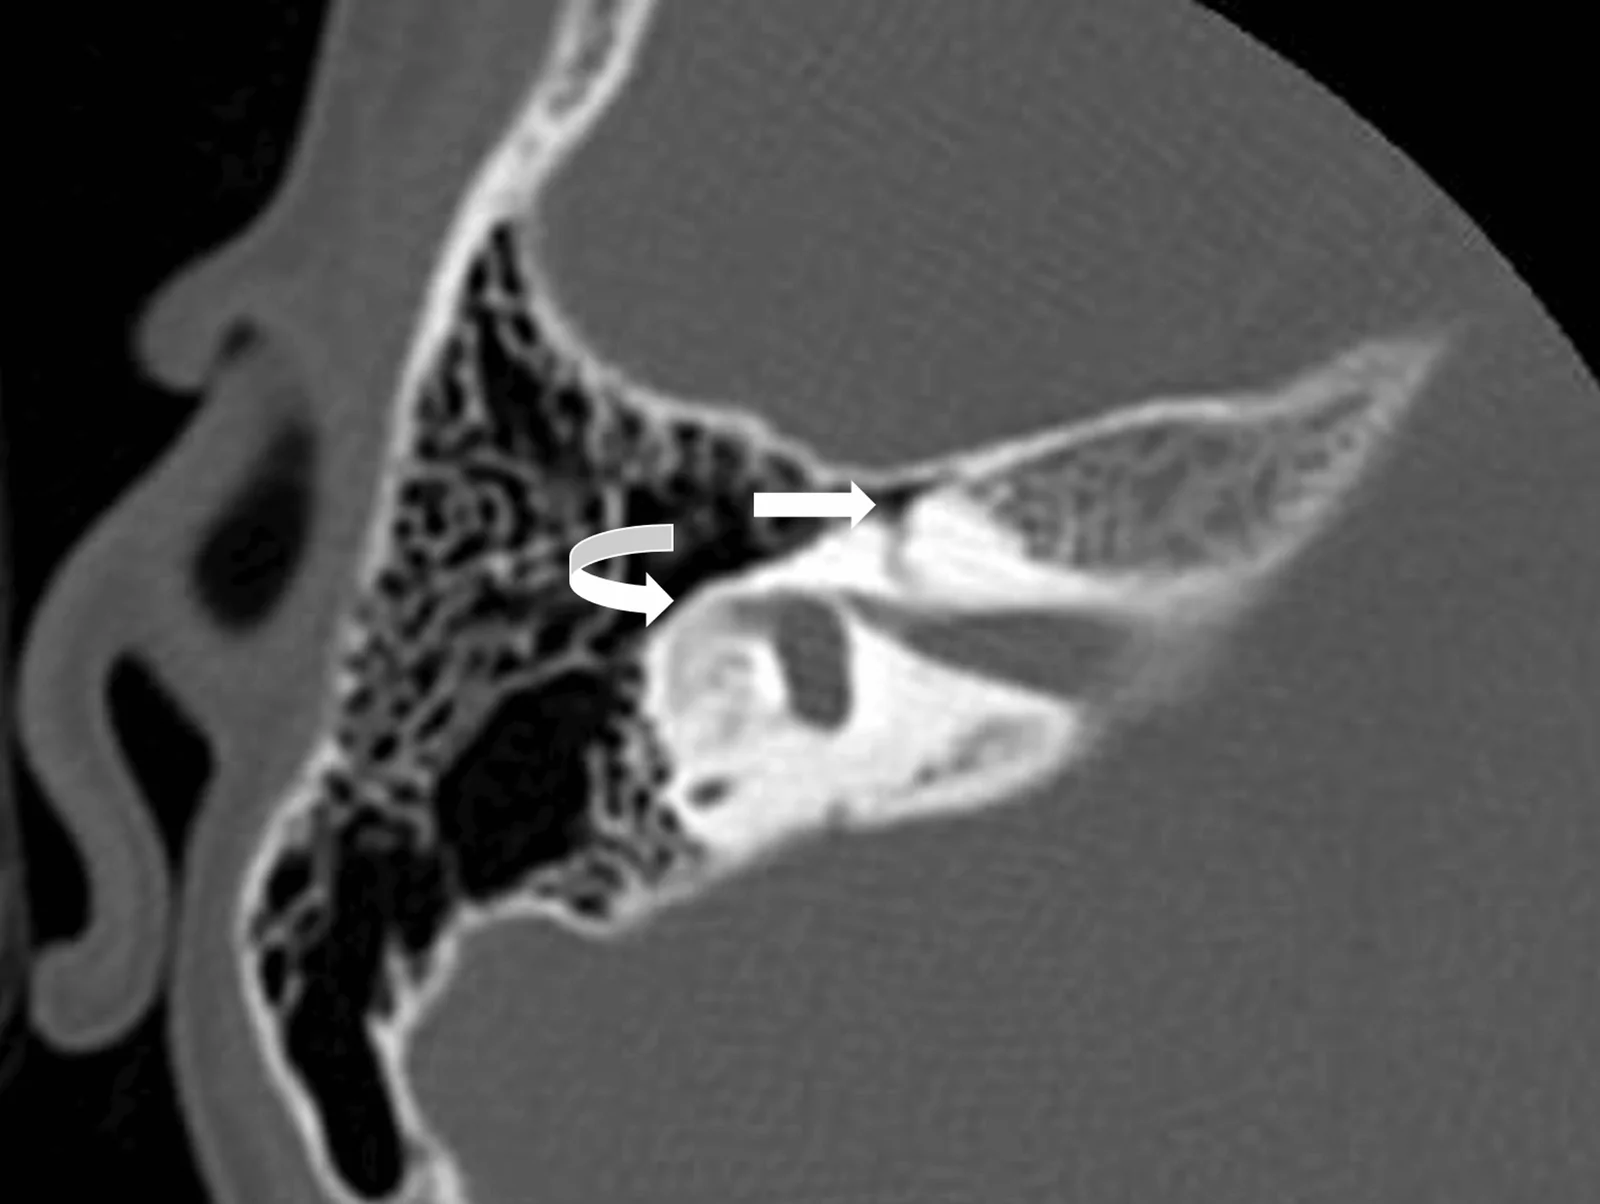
High-resolution computed tomography (HRCT) of the right temporal bone: axial section The images demonstrate a hypoplastic labyrinthine segment and severe hypoplasia of the tympanic segment of the facial nerve canal. Straight arrow: hypoplastic first genu and labyrinthine part of the facial nerve canal; curved arrow: severe hypoplasia of the tympanic part of the facial nerve canal
